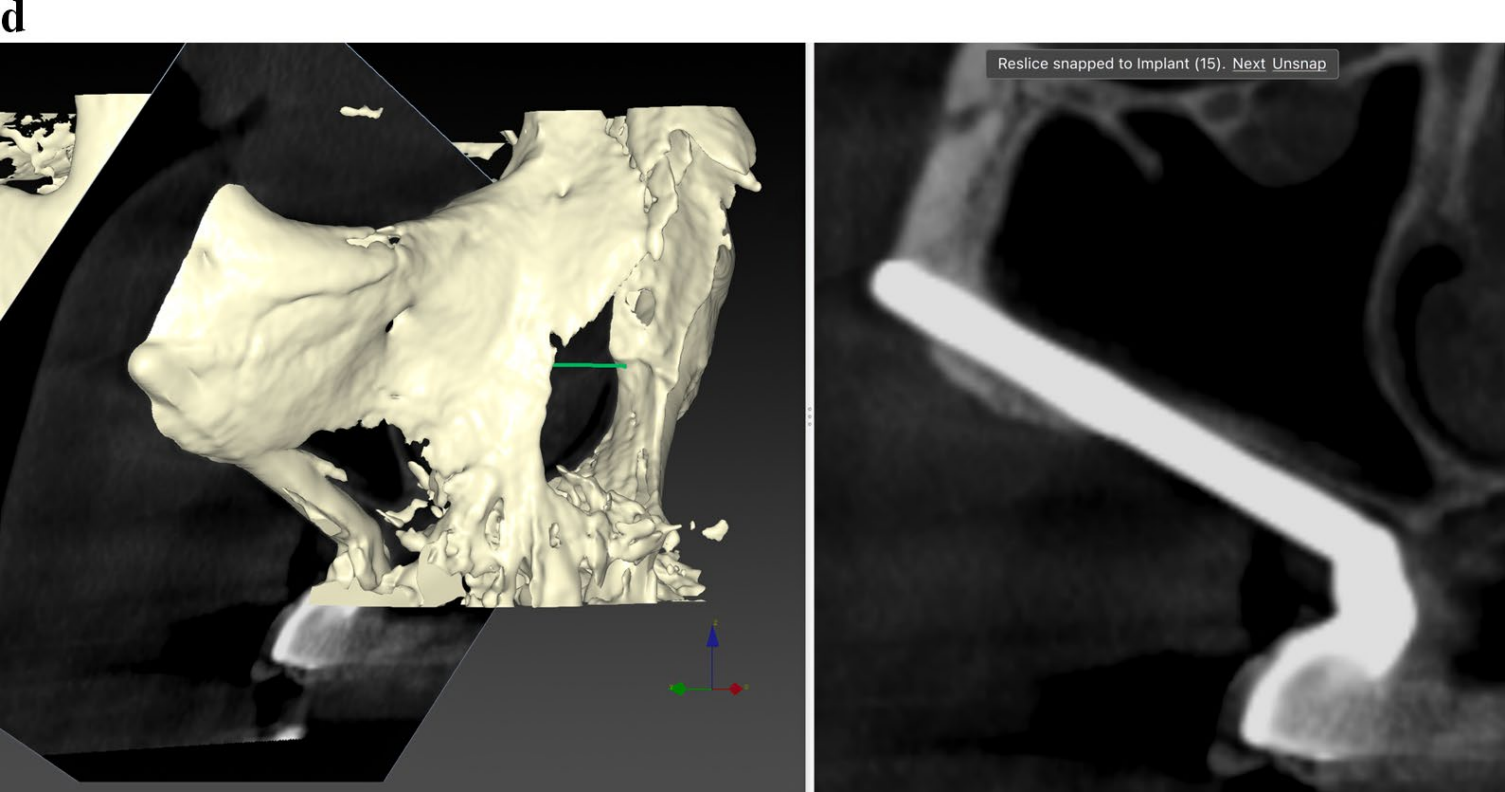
a Oblique CBCT slice taken on November 11th 2020. DTX Studio Implant software was used to plan the implant trajectory using the ZAGA Channel approach. b December 11th 2020. One week after surgery, planned in a, the patient presents with acute symptoms of rhinosinusitis. The oblique CBCT slice using DTX Studio Implant software shows complete opacity of the right maxillary sinus. c March 3rd 2021. Three months after surgery. After medical treatment, the clinician’s symptoms disappear, and when we compare the image of this figure with the one in Fig. 11a, we may verify that the M-LM is negative. d The DTX Studio Implant software allows us to superimpose a virtual implant on the real one and analyze it. The new CBCT was taken on December 22, 2023, 3 years after the surgery. When we compare it with the CBCT before surgery, we verify that the M-LM is negative
